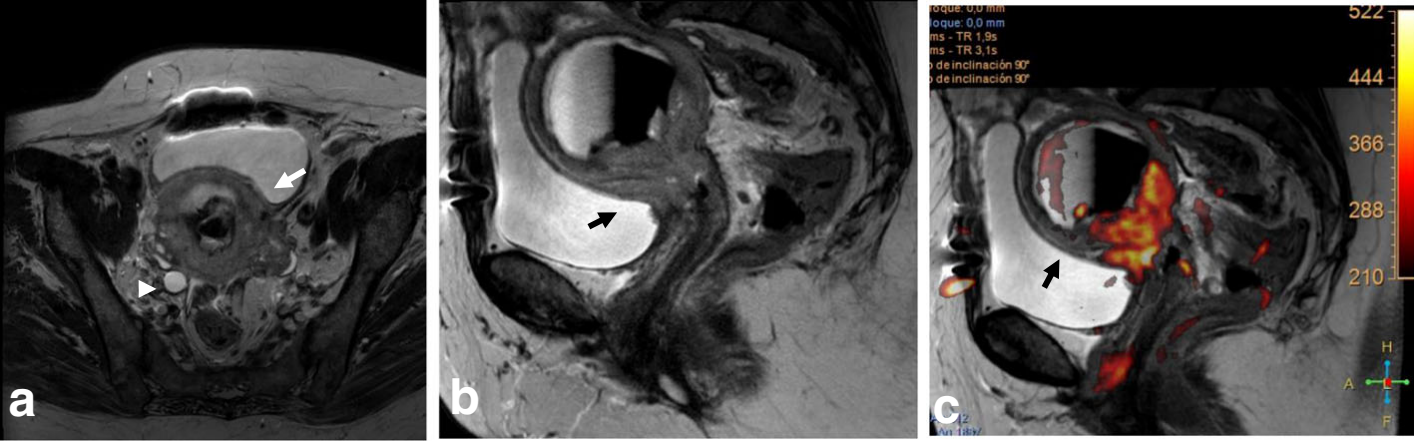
Cervical cancer. a Axial oblique T2WI shows a cervical mass (arrow), with hematometra and ureteral invasion (arrowhead) (IIIB). b Sagittal T2WI shows loss of the hypointense rim of the posterior bladder wall suggesting invasion (arrows). c T2WI+DWI reveals no invasion of the bladder wall (arrow)
